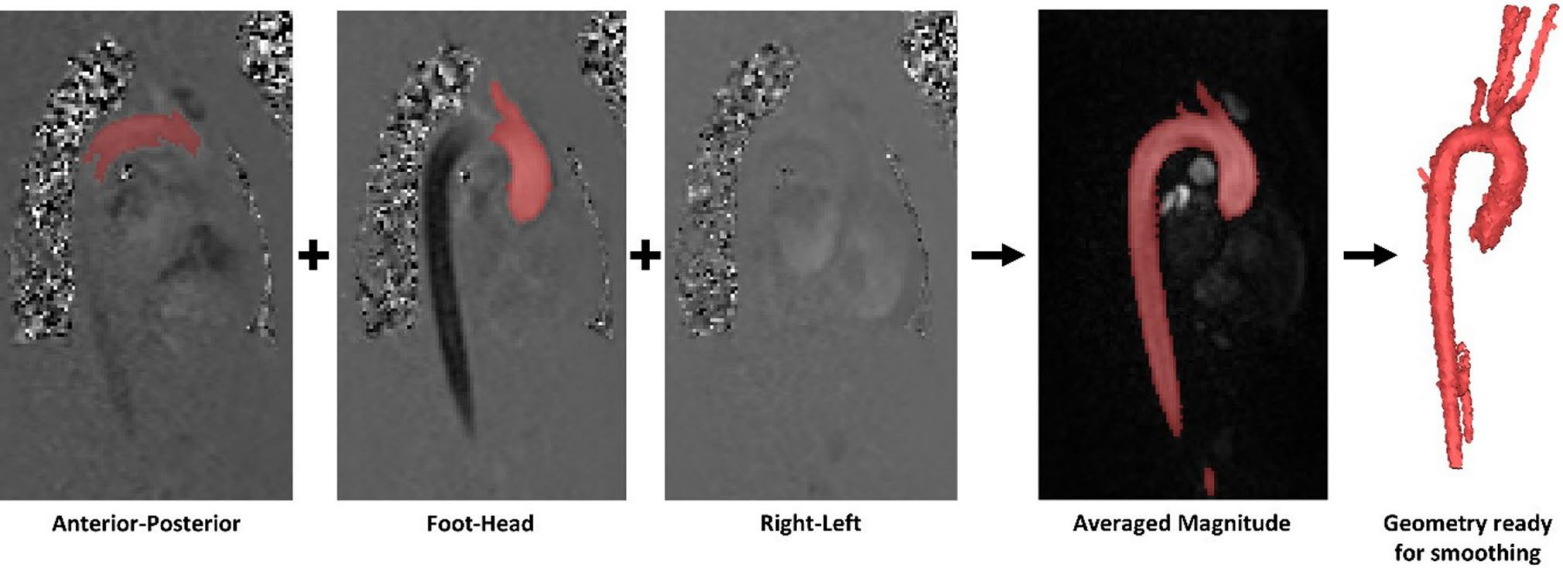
Reconstruction of healthy aorta from 4D flow MRI data. Segmentations from each velocity phase are combined on the magnitude image to form a complete 3D model
